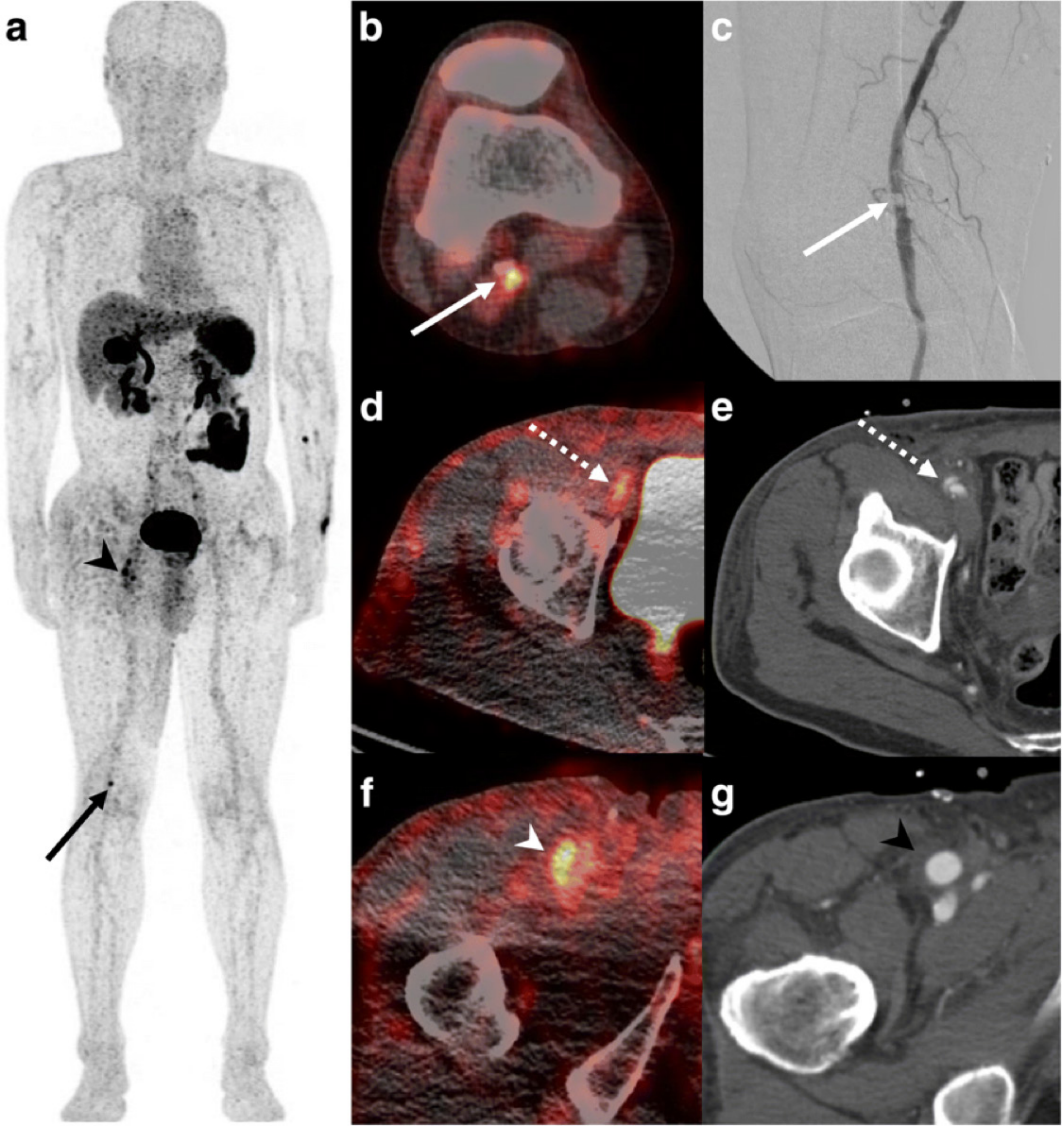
18F-GP1 arterial uptake in right popliteal artery. 18F-GP1 PET-CT images of a patient who had recently undergone right common femoral artery endarterectomy and right popliteal artery angioplasty. Anterior maximum intensity projection and axial images taken 120 min after 18F-GP1 injection show focal increased uptake in the right popliteal artery (a, b); arrows), which corresponds to a thrombotic lesion after angioplasty (c). Additional 18F-GP1 uptake is seen in the dissected right distal external iliac artery (d, e); dotted arrows) and right common femoral artery (a, f); arrow heads) where endarterectomy was performed 3 days prior to the PET-CT (g, arrow head). Images courtesy of Chae et al.^40^ PET, positron emission tomography.
